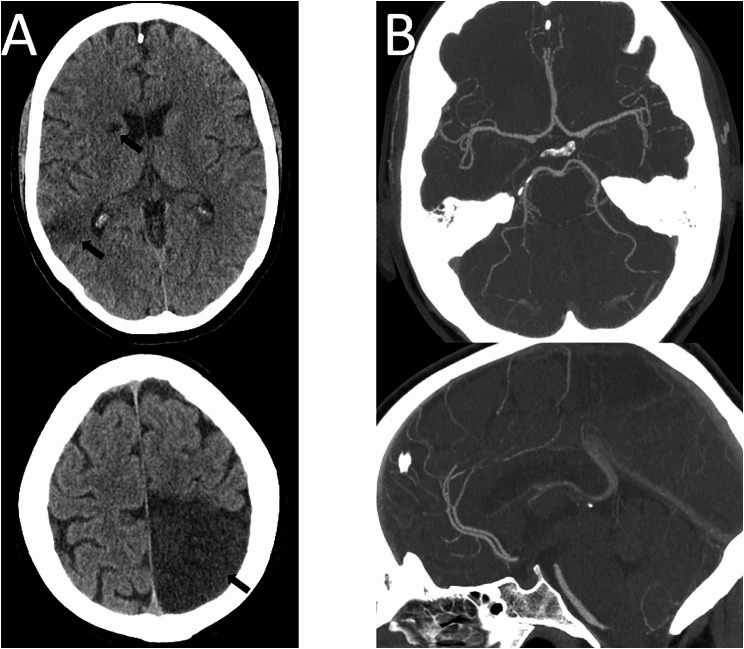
Follow up imaging A: Non contrast CT axial slices showing established infarcts in the right caudate, right temporoparietal and left parietal regions (arrows) B: CT angiogram MIPs axial (top) and sagittal (bottom) showing complete resolution of previous multifocal stenoses
